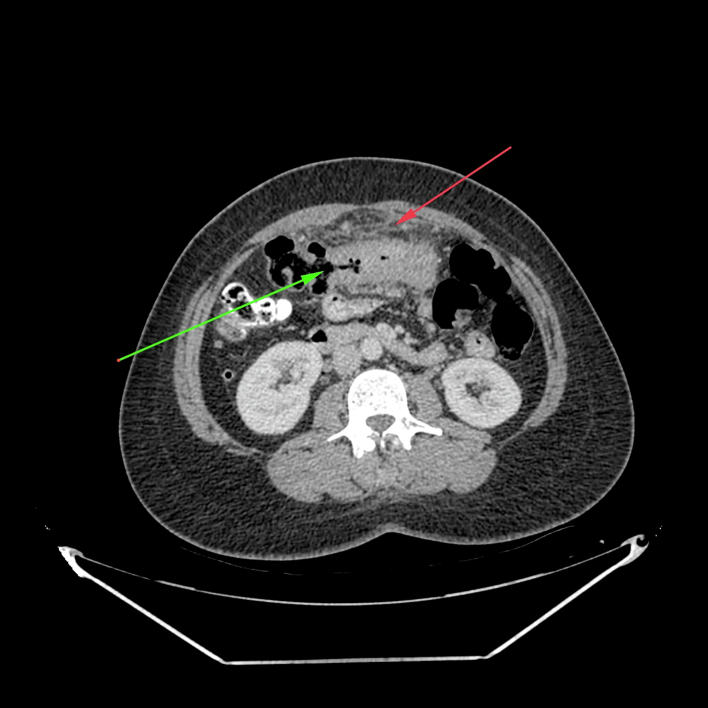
Axial CT view showing luminal mass lesion demonstrating shouldering “Apple core” deformity (green) with surrounding inflammatory infiltration into anterior abdominal wall (red). (For interpretation of the references to colour in this figure legend, the reader is referred to the web version of this article.)
